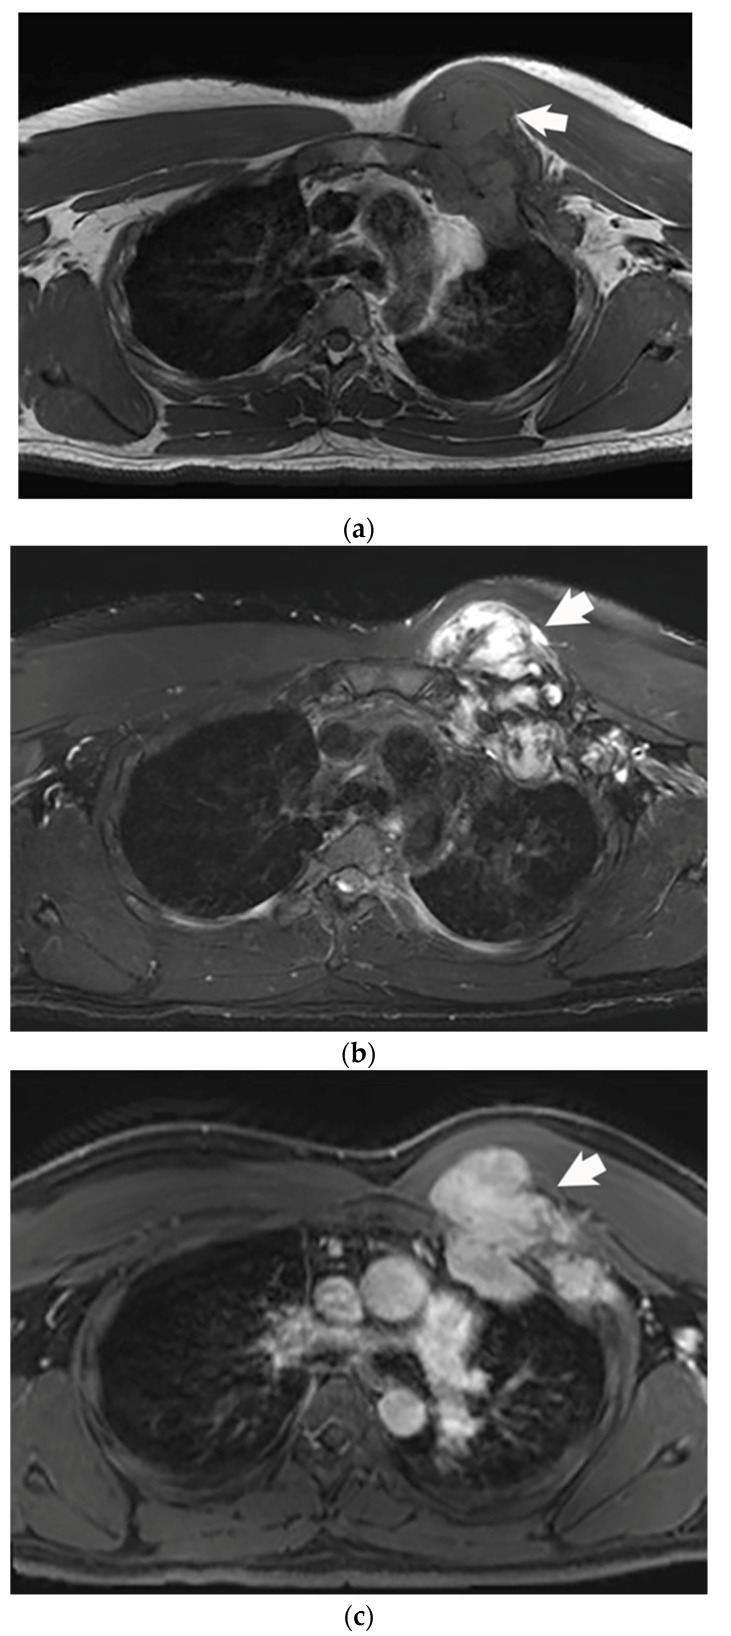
45 year old male with a spontaneous, extra-abdominal desmoid tumor (arrow) involving the left chest wall. (a) A selected pre contrast T1 magnetic resonance imaging image demonstrating iso to hypo intensity of the desmoid tumor as compared to the skeletal muscle. (b) Selected magnetic resonance imaging image demonstrating predominantly hyperintensity of the desmoid tumor (arrow) on a fat saturated T2 sequence. (c) Selected magnetic resonance image demonstrating avid enhancement of the desmoid tumor (arrow) after administration of gadolinium based intravenous contrast.
